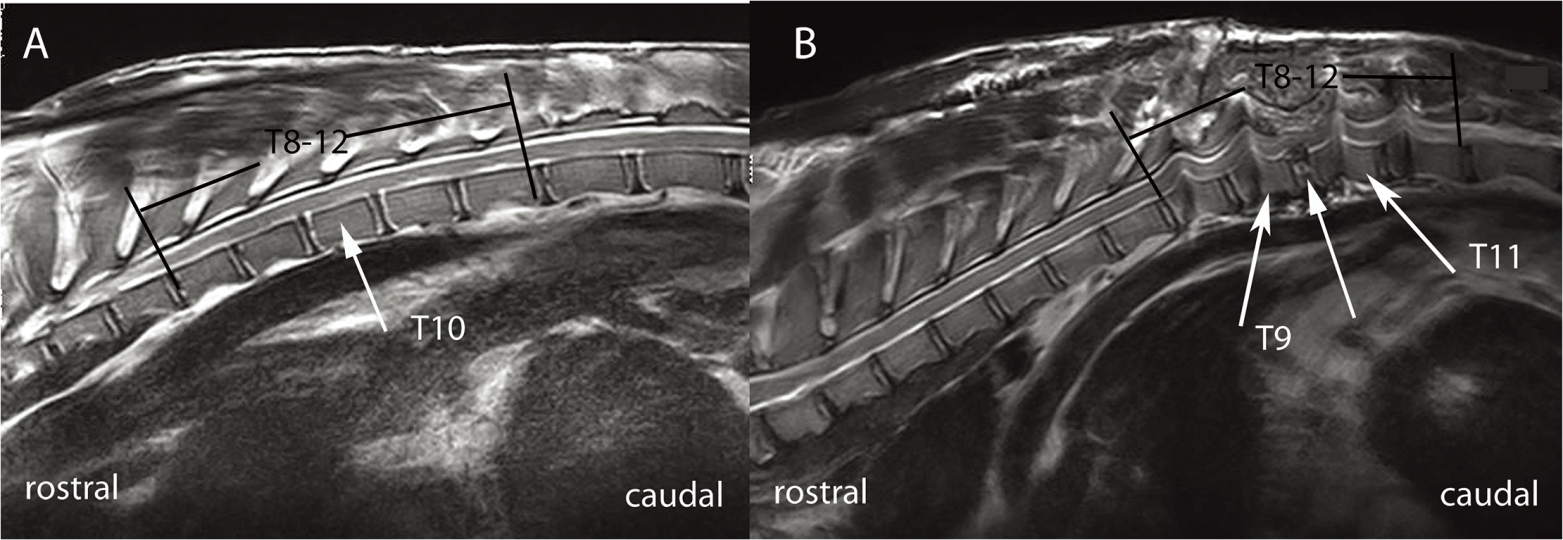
Pre- and postoperative magnetic resonance images (MRIs) of T9-11. (A) Preoperative MRI showing a normal signal at the T8-12 spinal cord. (B) MRI three days postoperatively showing successful complete resection of the T10 vertebral body and adjacent discs and a normal signal at the T8-12 spinal cord.
